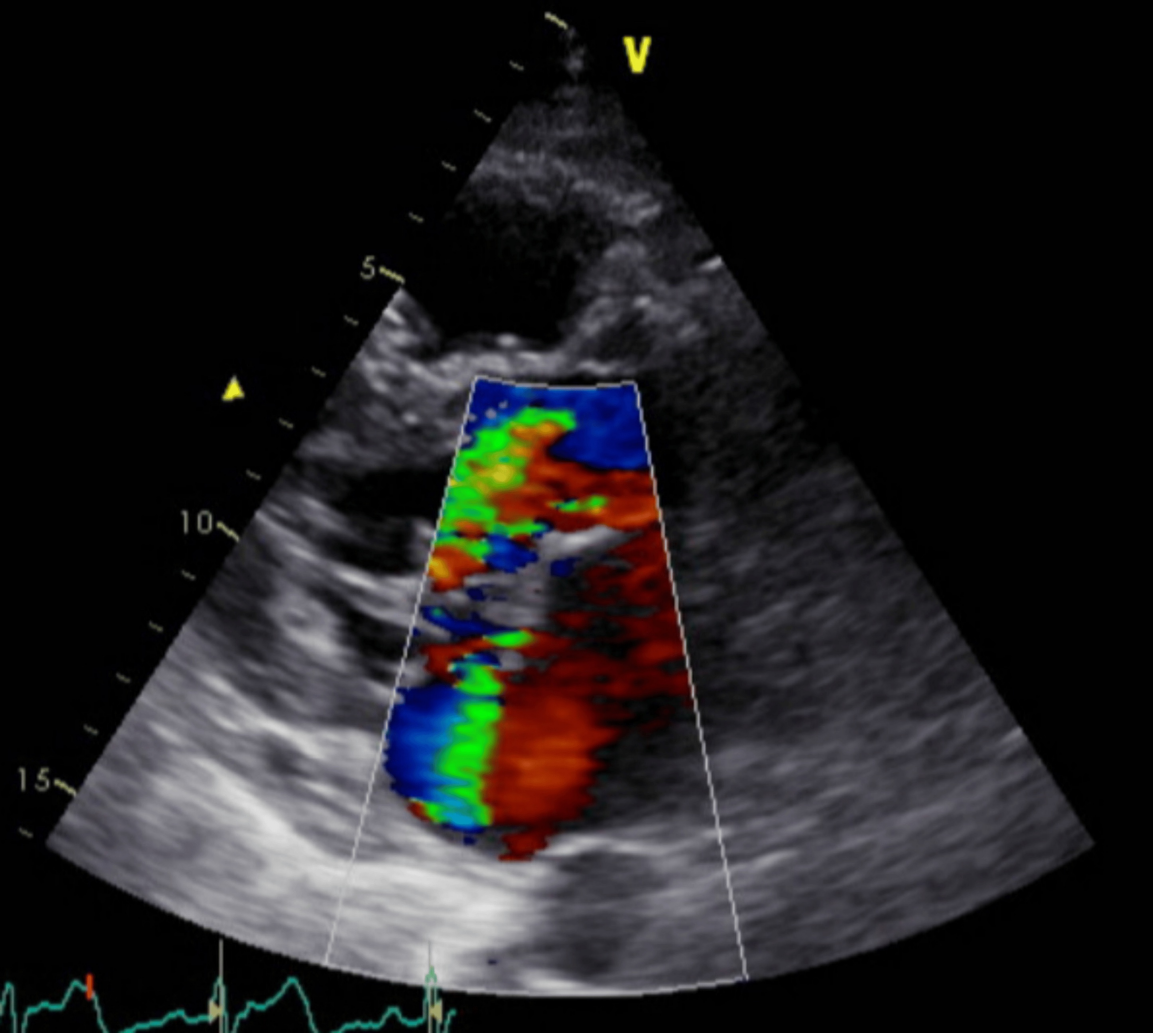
Color Doppler of parasternal long axis showing Mitral Regurgitation
